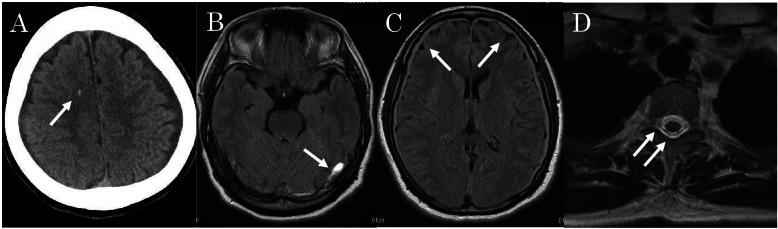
a Axial cranial CT showing microbleeds in the right frontal lobe (arrow). b Axial cranial MRI showing subdural hematomas (arrow). c Axial cranial MRI with contrast showing thrombosis in the left transverse and sigmoid sinuses and narrow lateral ventricle (arrows). d Postmyelography CT showing a cervical CSF leak into the epidural space (arrows). CSF, cerebrospinal fluid; CT, computed tomography; MRI, magnetic resonance imaging
